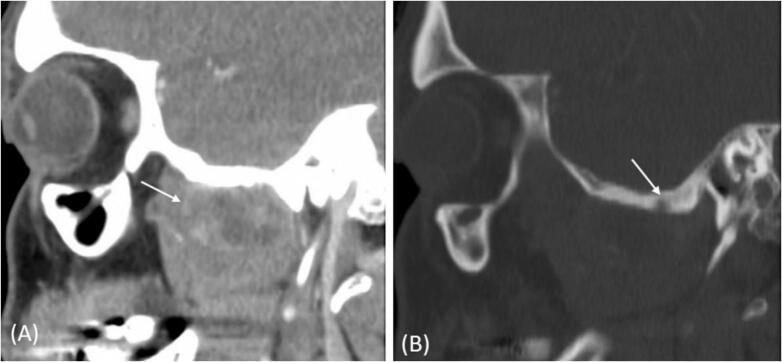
(A) CT scan post contrast with sagittal view in soft tissue reconstruction shows the soft tissue mass (arrow) in right ITF with heterogeneous low density and faint peripheral calcifications. (B) CT scan with sagittal view in bone algorithm reconstruction shows the sclerosis of the temporal bone with erosion of the outer cortex (arrow).
